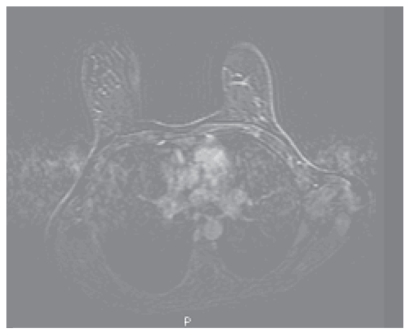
Dynamic contrast enhanced Breast MRI following 6 cycles Docetaxol shows no residual abnormal enhancement and complete resolution of the previous highly suspicious, typically malignant MR findings.
